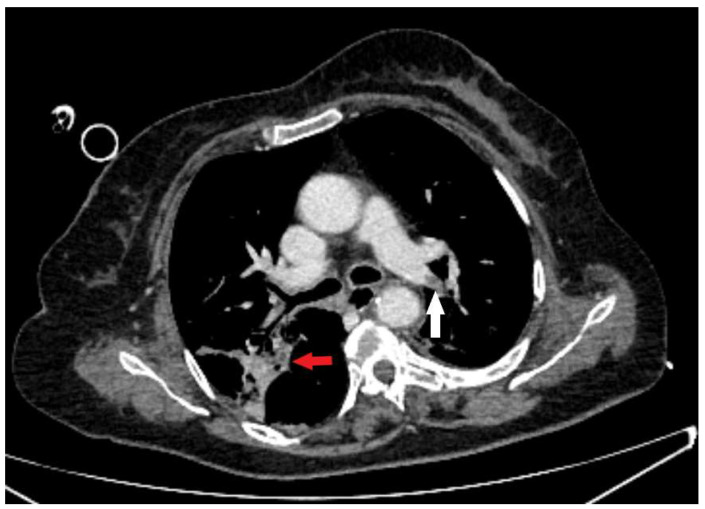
An axial CT scan demonstrates intraluminal defect in the postcontrast opacification of the lobar branch of the left pulmonary artery (white arrow), which corresponds to pulmonary thromboembolism with present consolidative parenchymal zones consistent with aspiration pneumonia (red arrow).
